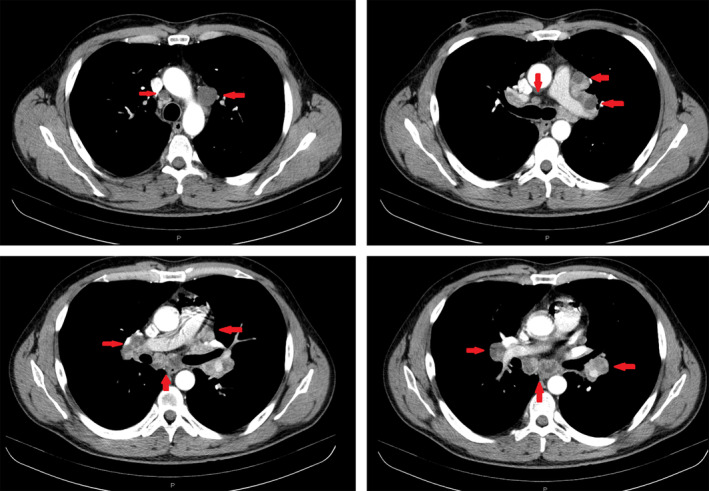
CT images showing the presence of abnormal mediastinal and hilar lymph nodes (red arrows).
